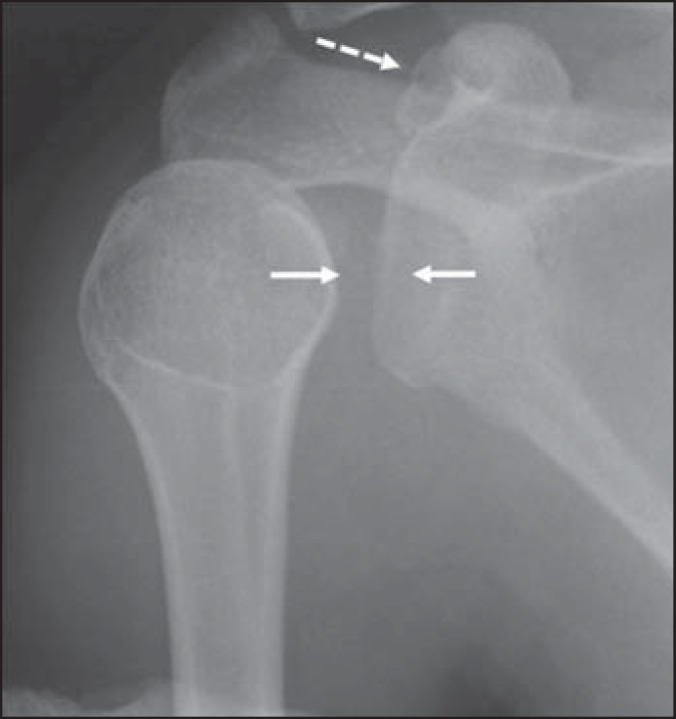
Radiography of right shoulder. Anteroposterior view showing glenohumeral
